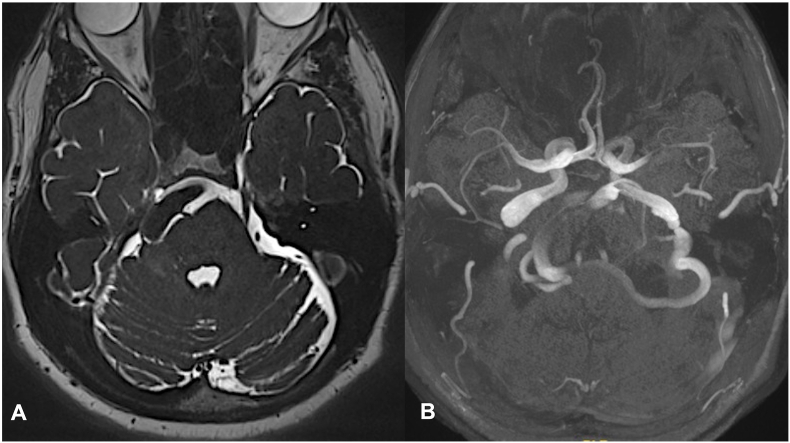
A. Axial T2-weighted magnetic resonance image demonstrating a prominent basilar artery ventral to the brainstem. The artery appears to be compressing the anterolateral aspect of the right side of the pons, near the expected location of the root entry zone of the right trigeminal nerve. B. 3D time-of-flight MR angiography showing a dilated and tortuous vertebrobasilar artery system. The vessel is deviating and curving laterally toward the presumed root entry zone of the right trigeminal and facial nerves, which may contribute to neurovascular compression.
